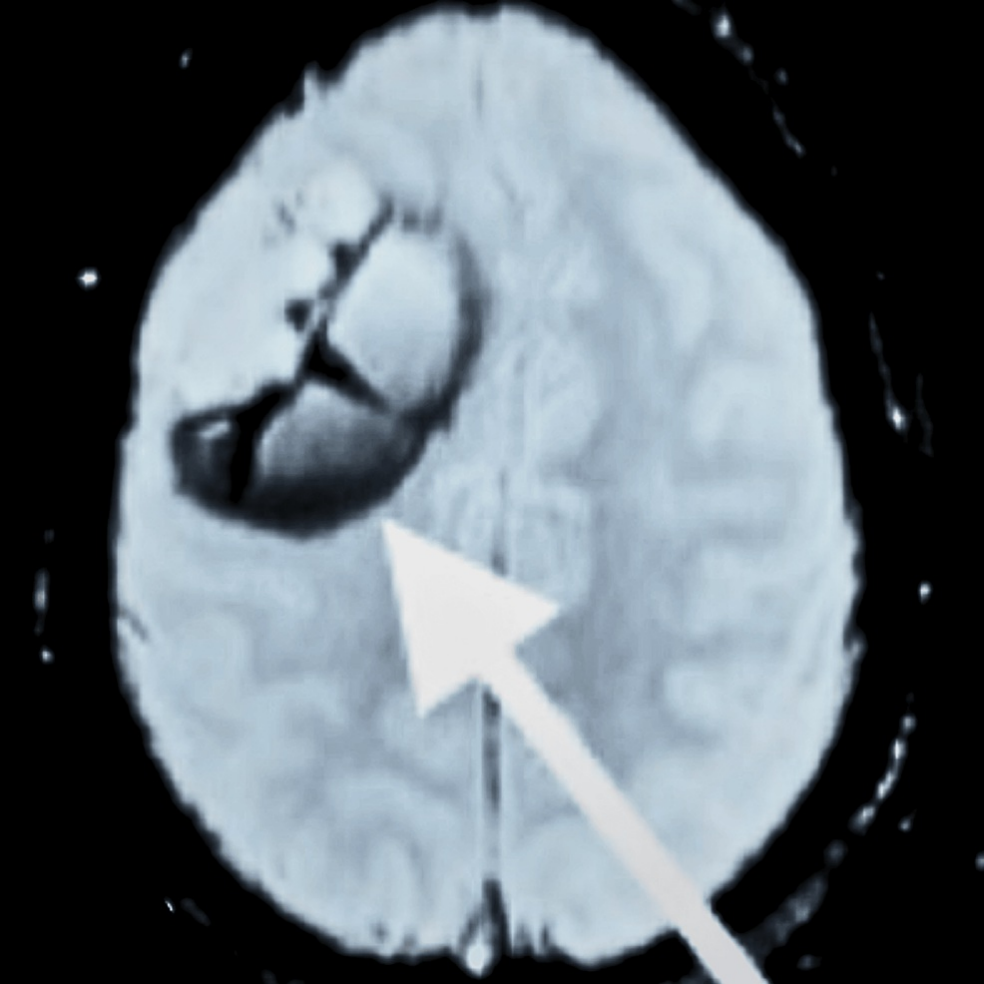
MRI of the brain The white arrow shows a well-defined, space-occupying lesion MRI: magnetic resonance imaging
